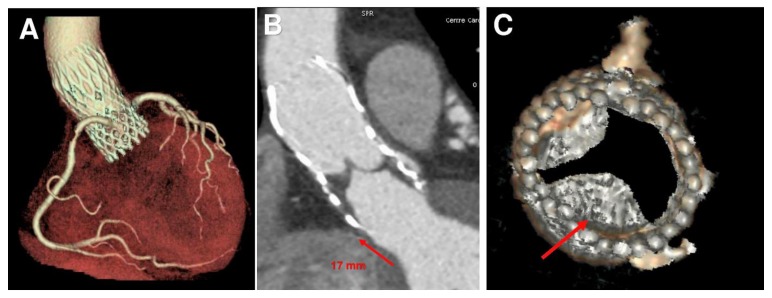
Transcatheter aortic valve replacement (TAVR) position, thrombus formation and biomechanical model of patient-specific aortic root from medical CT images. (A) Pre-operative CT scan of CoreValve (26 mm). (B) Post-operative CT scan at one year shows a device upward shift. (C) Thrombus formation in subvalvular zone (red arrow).
